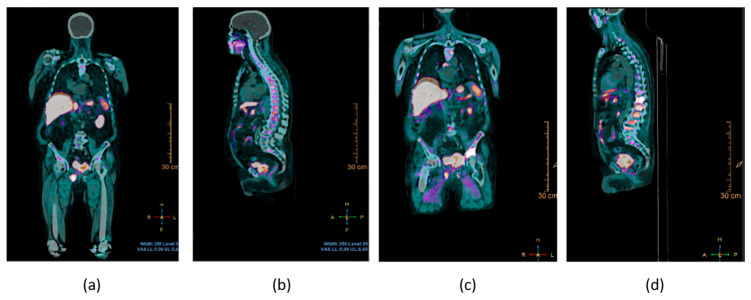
Male patient, 81 years old, with metastatic prostate cancer, choline PET-CT fusion images showing multiple bone lesions, coronal (a,c) and sagittal (b,d) reconstructions. Prostate cancer is the most common cancer in men; it can give bone metastasis but, due the osteosclerosing nature of these lesions, rarely requires orthopaedic surgery.
